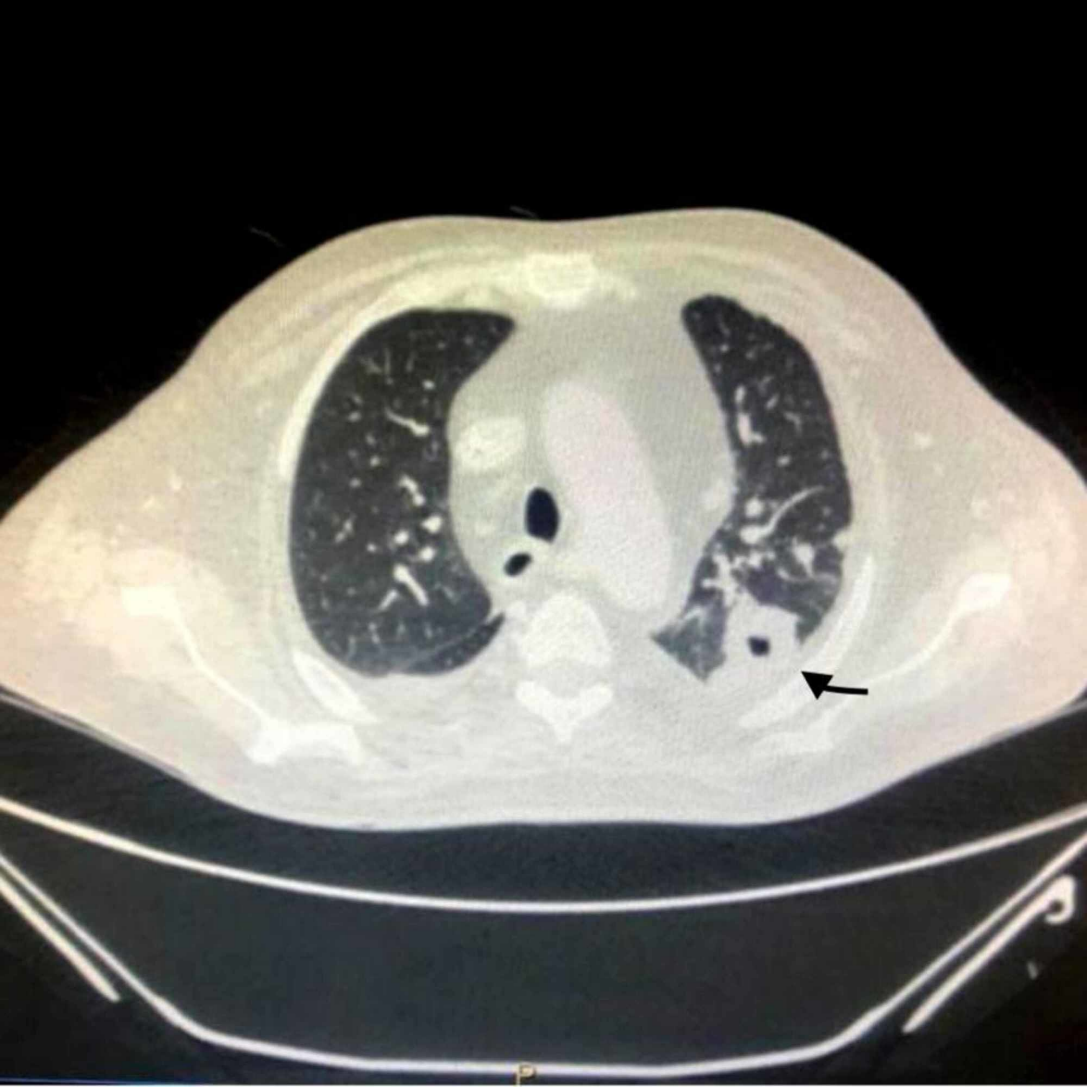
CT scan showing subpleural ground glass opacity and cavitation demonstated by black arrow.
